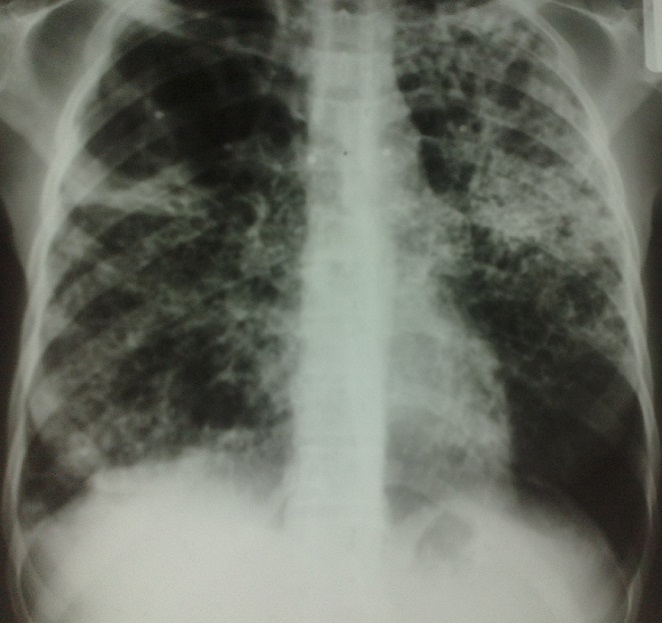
Radiographie du thorax, lésions pulmonaires excavées étendues et bilatérales chez un BPCO
